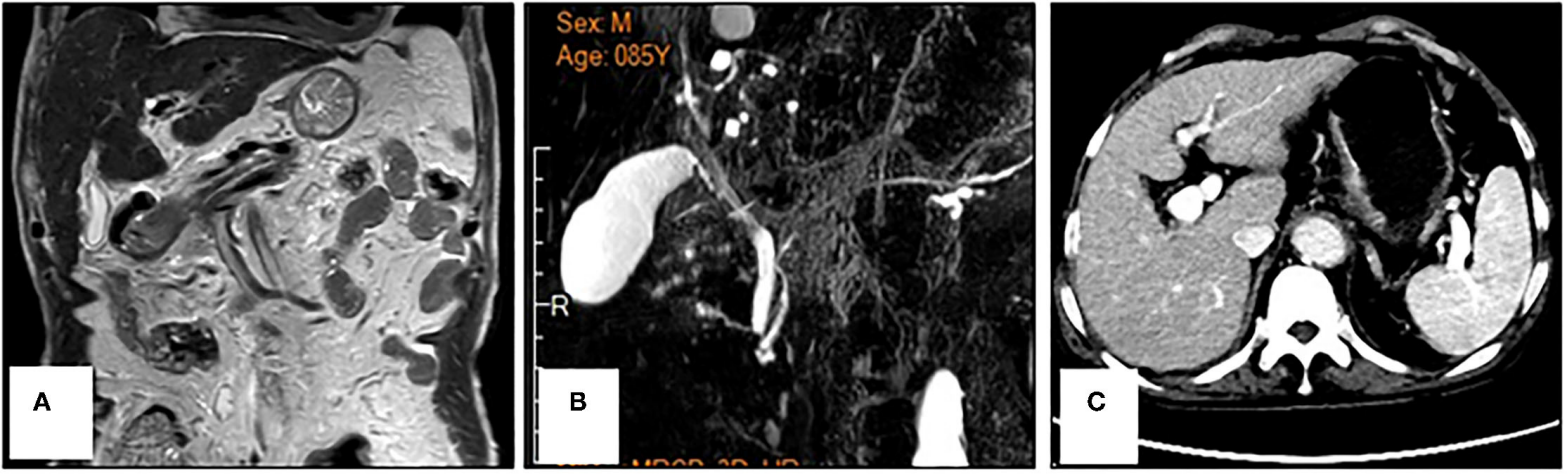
Reprensentative images of magnetic resonance cholangiopancreatography (A,B) and abdominal computed tomography (C) in a patient with metastatic gastric cancer and rare severe immune-related cholestatic hepatitis and subsequent severe late-onset pneumonia after pembrolizumab therapy. No obvious biliary obstruction was observed.
